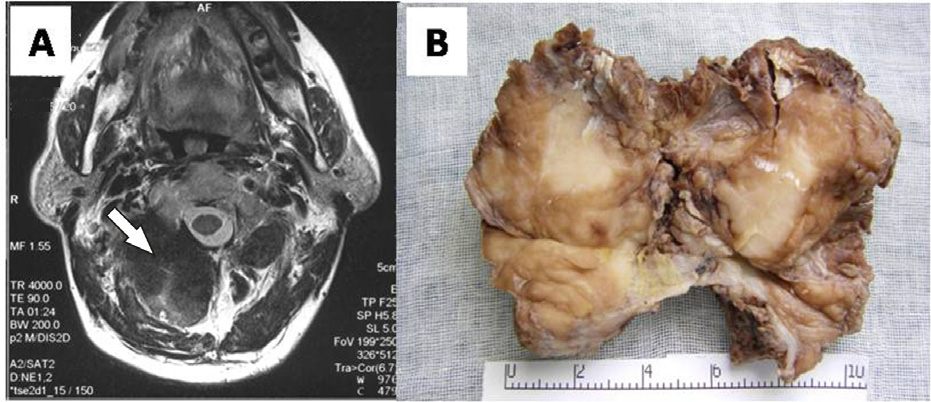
Views of the tissue mass. (a) Magnetic resonance imaging scan. A soft-tissue mass is seen in the right shoulder (white arrow). (b) Gross morphology.
